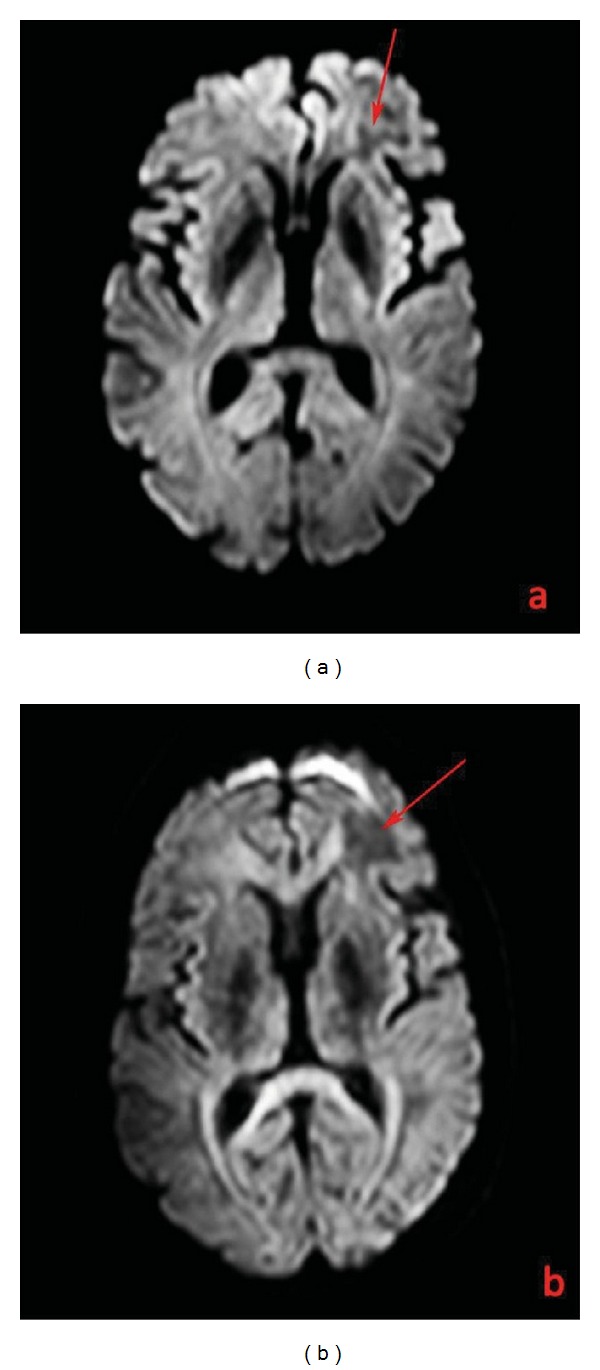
DWI ADC map (Philips Achieva 3T, TR = 4500 ms; TE = 112 ms) in December 2011 (a) and May 2012 (b) of the white matter lesion underneath the frontal lobe of the left hemisphere (arrows) with some diffusion restriction in a 44-year-old man with a 12-year history of MS affected by natalizumab-related PML.
